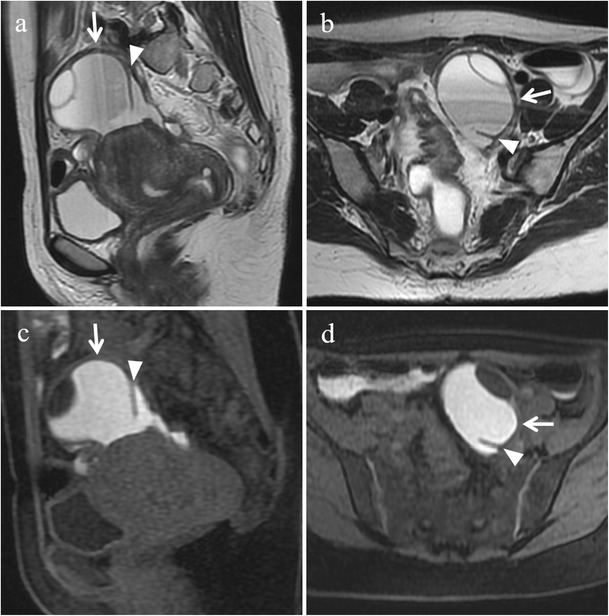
Hematosalpinx in a 46-year-old woman with endometriosis. The same patient as in Fig. 12. (a) Sagittal and (b) axial T2-weighted images show a tortuous, tubular structure with internal fluid-fluid level in the left adnexa (white arrows). On (c) sagittal and (d) axial fat-suppressed T1-weighted images endoluminal content displays high signal intensity (white arrows), a finding consistent with hematosalpinx. Note the incomplete mucosal and submucosal plicae along the tubal wall (white arrowheads)
